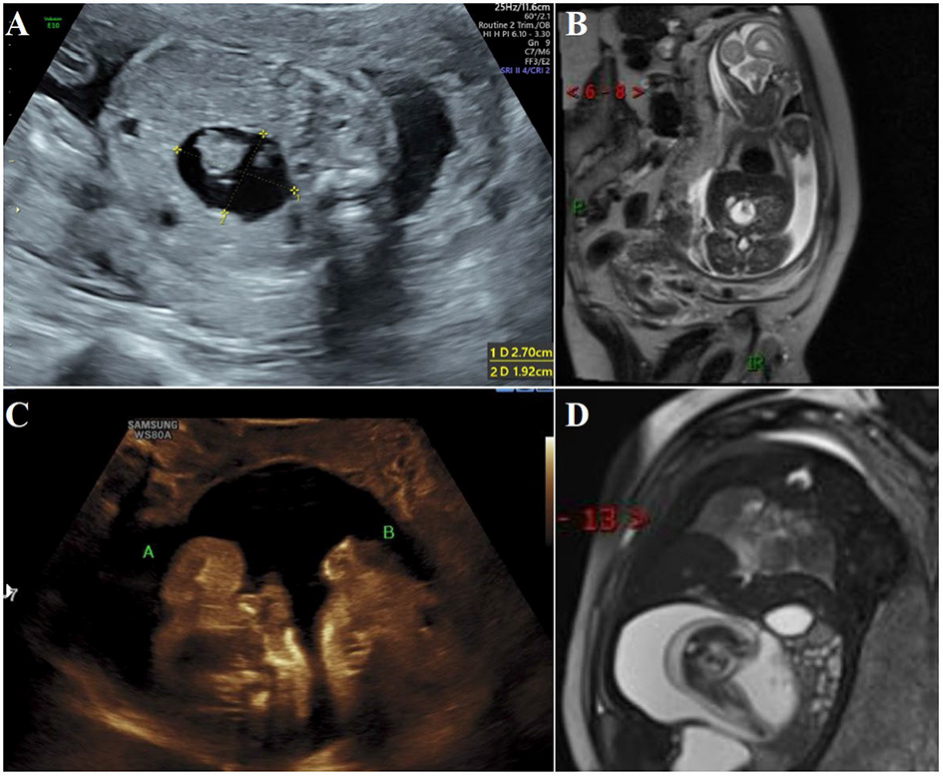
During antenatal examination, the parasitic fetus was found by ultrasound and MRI. (A) Ultrasound examination at 22 gestation weeks showed mixed density shadow of the right upper abdomen of the fetus; (B) MRI at 22 gestation weeks showed a long T1 long T2 signal foci measuring 2.4 × 1.9 cm near the midline area of the right upper abdomen of the fetus. The boundary was clear and the shape was regular. There are short cord-like short T2 signal foci in the lesion; (C) At the ultrasound examination at 37 gestation week, two parasitic fetuses were found; (D) MRI at 37 gestation weeks showed a round long T1 long T2 signal focus measuring 11.1 × 8.7 × 6.4 cm near the midline area of the right upper abdomen of the fetus, with clear boundaries and regular morphology. Fetal structures can be seen in the lesion, and adjacent tissues are compressed.
